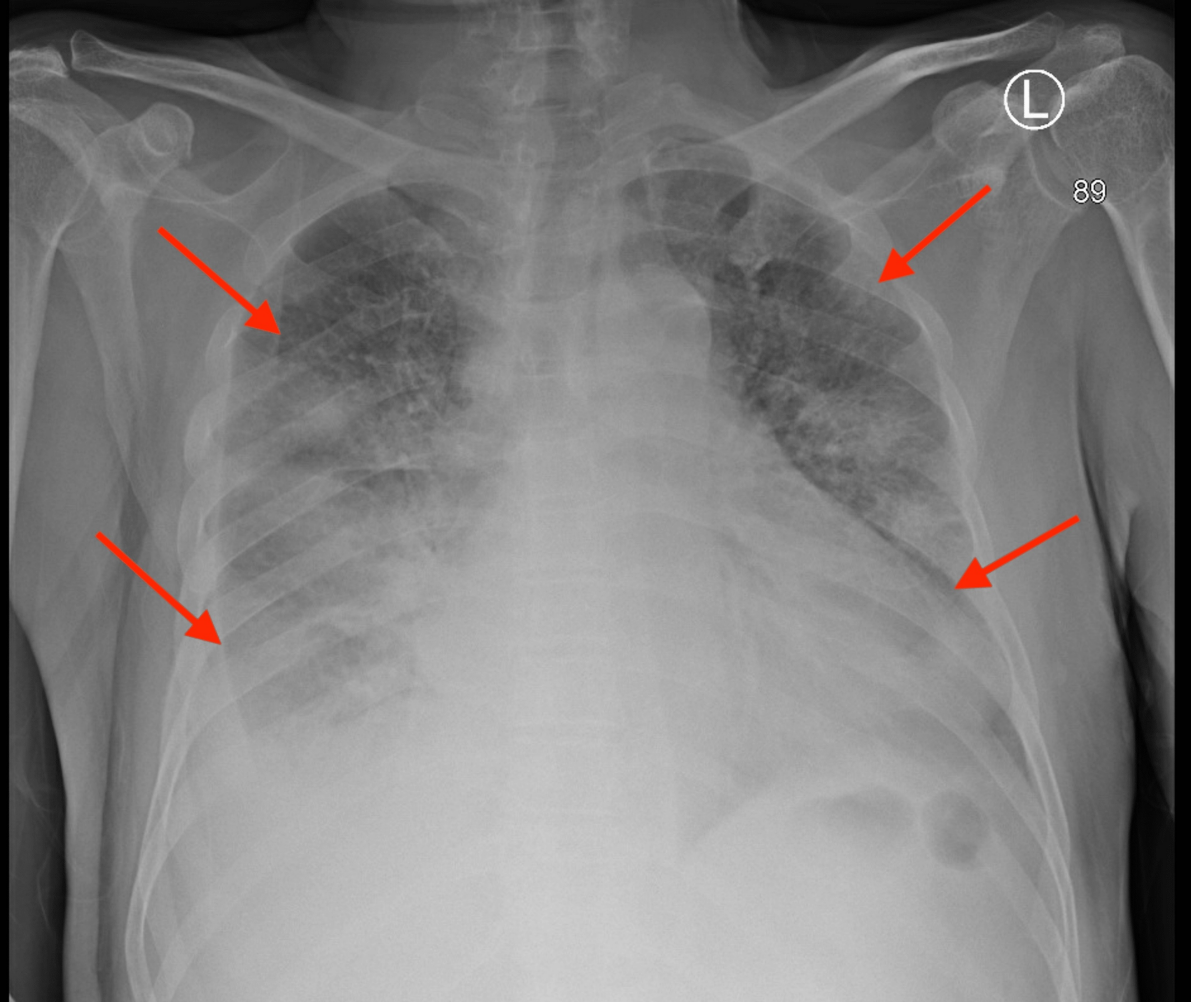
Chest X-ray revealed bilateral peripheral and basilar infiltrates along with cardiomegaly.
